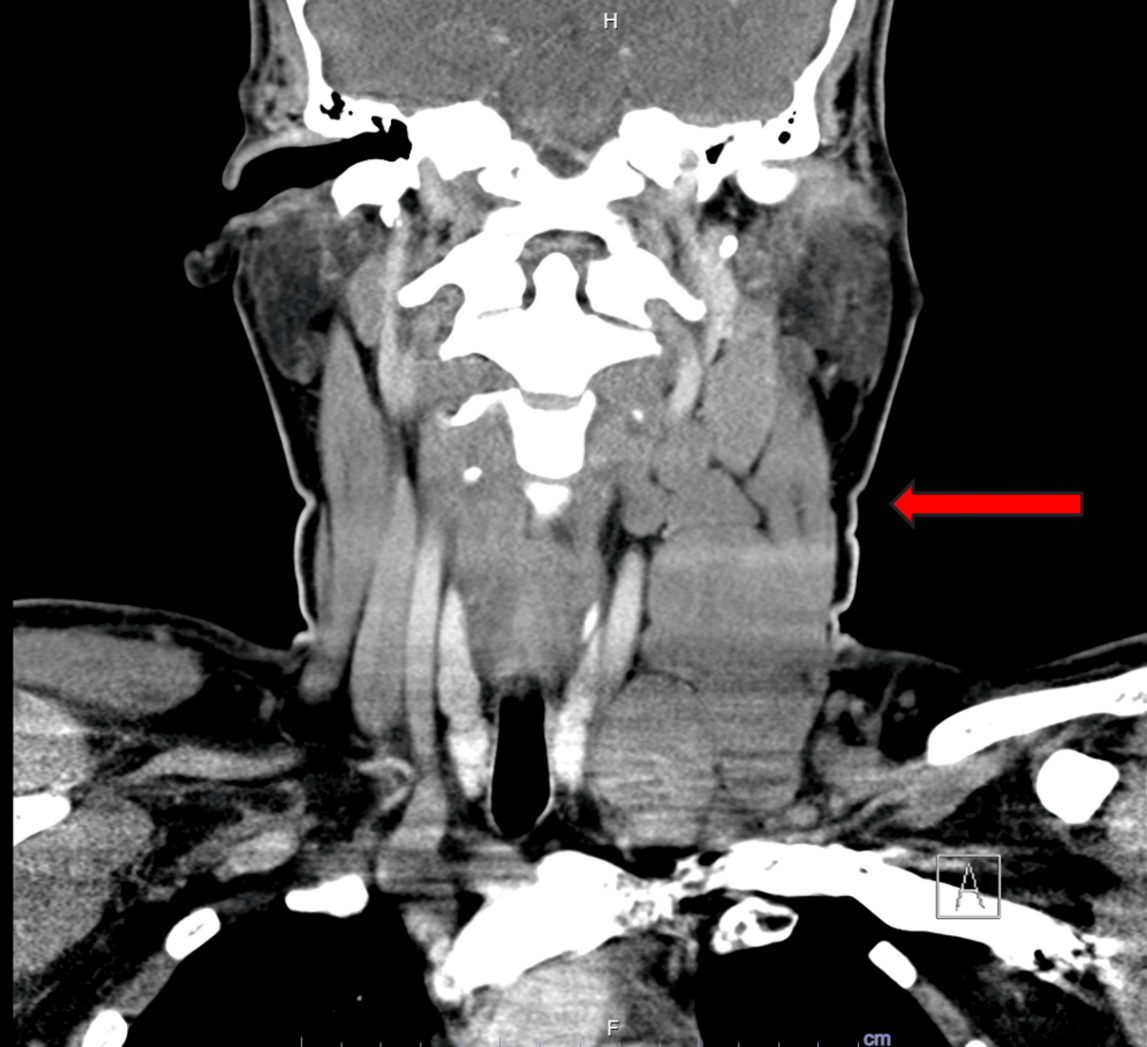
Coronal view of CT neck The red arrow highlights the left cervical lymphadenopathy.
